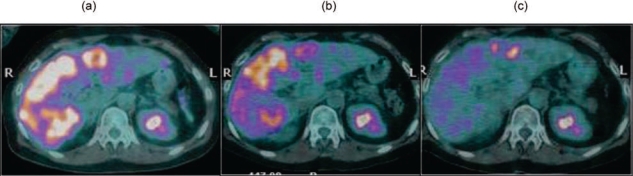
(a) basal CT-PET performed before SIR-Spheres therapy in patient with metastases from breast cancer shows huge masses into the hepatic right lobe; (b) CT-PET two months after treatment confirmed FDG uptake reduction; (c) CT-PET three months after treatment showed a very minimal residual tumour tissue still alive
